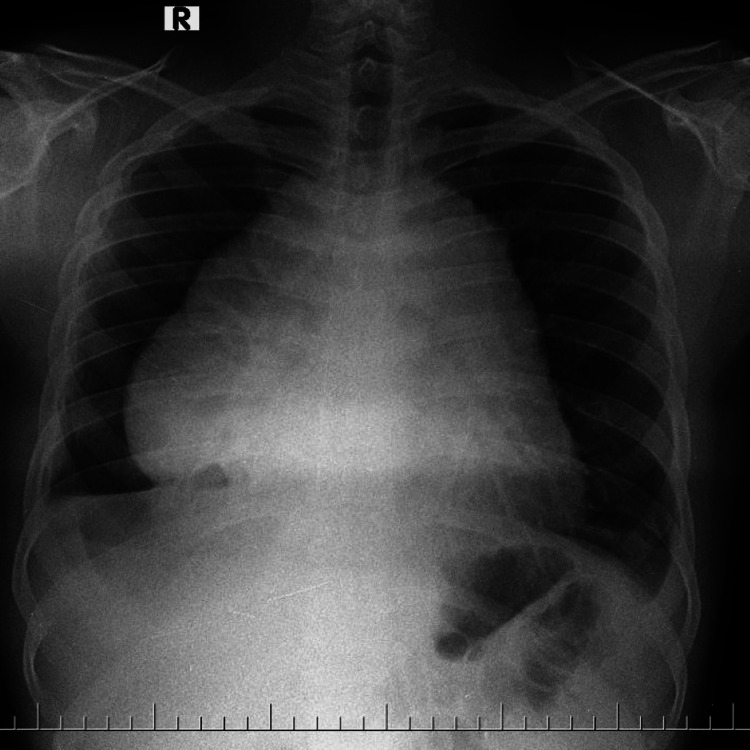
A post-thoracentesis chest radiograph revealed an expanded lung. Also, evidence of mediastinal widening with the hilum overlay sign and cardiomegaly was noted.
